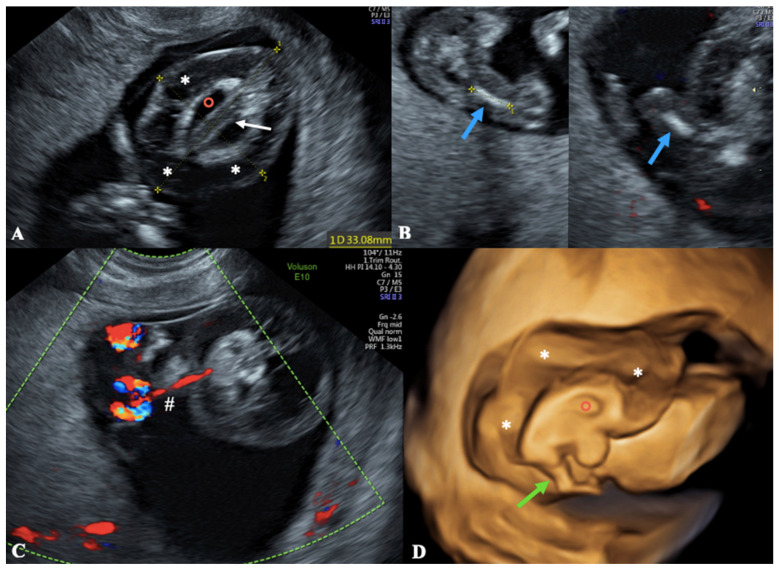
A transvaginal ultrasound in a 12-week pregnant patient. (A) The monster twin appeared as a hypo-hetero echoic mass with a rudimental vertebral column (white arrow) behind an anechoic cyst (red circle), and marked generalized edema of soft tissues (*). (B) An axial view of the monster twin showing femoral bones (blue arrows). (C) An Axial view of the monster twin highlighting retrograde umbilical perfusion (#) and absence of the heartbeat. (D) A sagittal view of the acardiac with 3D ultrasound technique showing anatomic details more clearly than the 2D standard way (the green arrow points to the sketch of a lower limb; * indicates the marked generalized edema of soft tissues).
